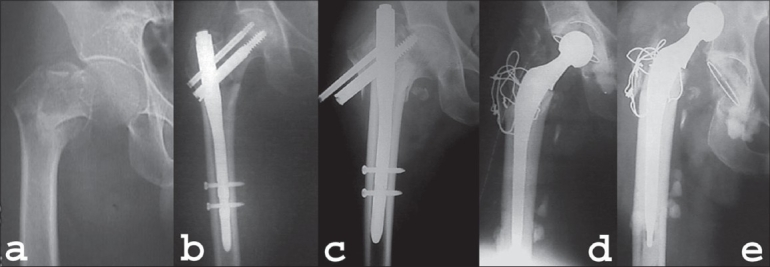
(a) AP radiograph of 70 year old patient showing intertrochanteric fracture of right hip. (b) Fracture was fixed with Proximal femoral nail. (c) Fracture collapse with screw back out three months after the surgery. (d) PFN was removed and cemented total hip arthoplasty was performed and trochanteric wiring was done. (e) Patient had dislocation of his hip two weeks after replacement surgery which was managed conservatively
